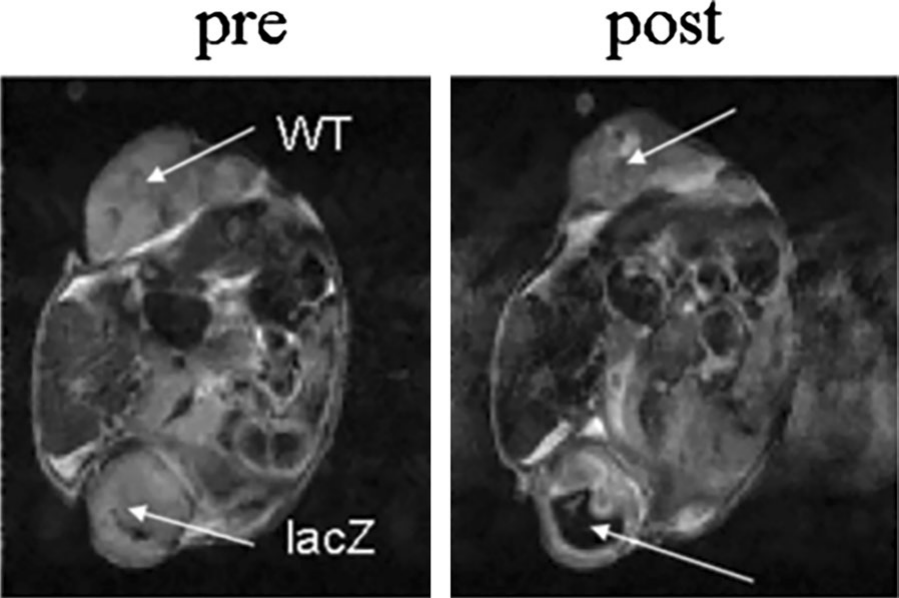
MRI detection of beta b-gal activity in MCF breast tumor transfected with lac Z. After intratumoral injection of S-Gal and ferric ammonium citrate, the tumor expressing lac Z shows strong hypointense contrast [111]. Reproduced with permission from John Wiley and Sons and Cui et al. [111]
